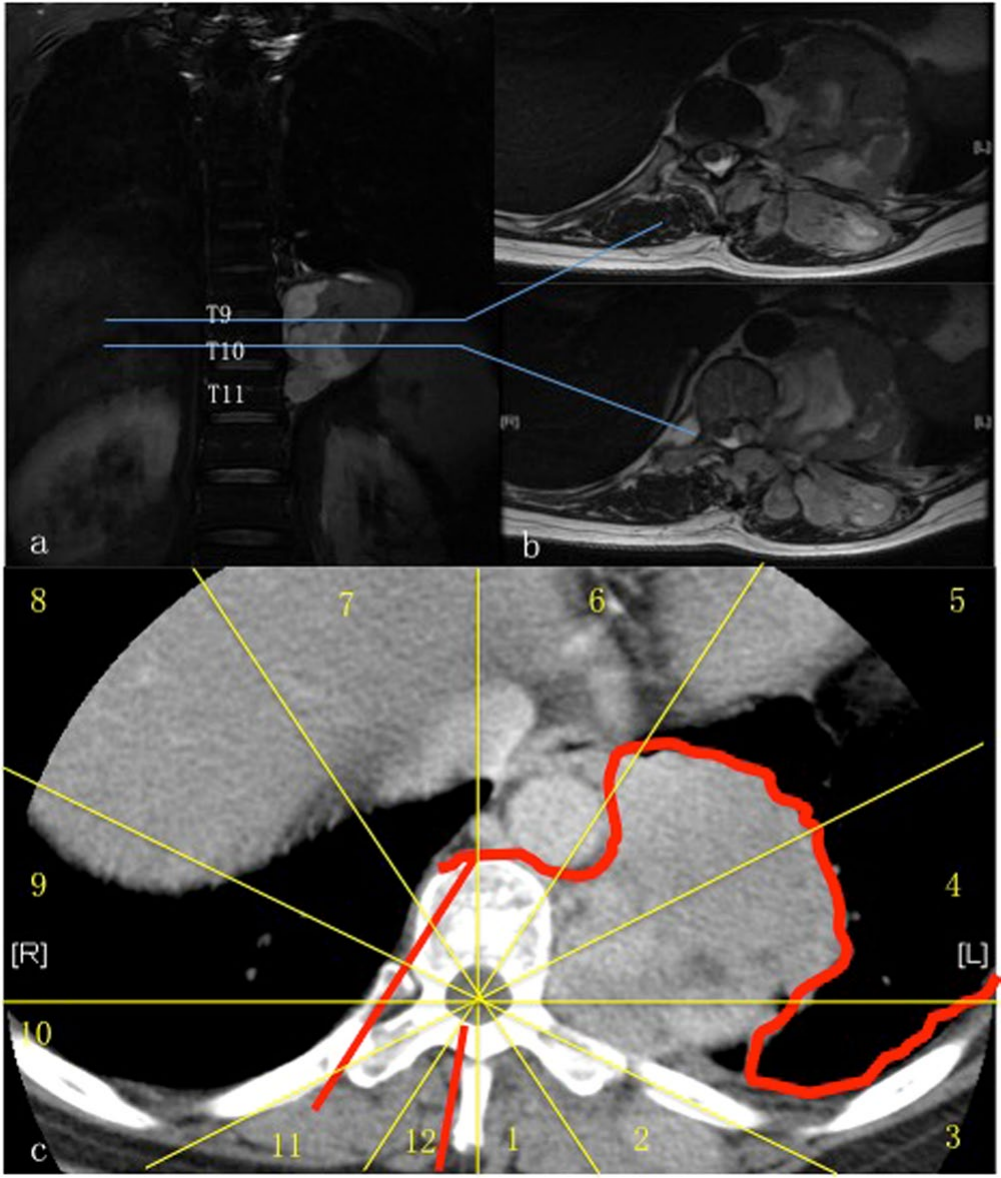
A case of a 63-year-old female with solitary fibroma at T9–11. The patient complained of numbness in the back and the abdomen 7 years after undergoing a piecemeal resection of a primary tumor in the paravertebral region of the lower thoracic spine in another hospital. Postoperative pathological diagnosis after initial surgery was solitary fibroma. a-c. MR images (a-b) and CT scans (c) show tumor recurrence along the left side of T9 to T11 vertebra (zone 1–5, A-D, WBB). Sagittal en bloc resection was planned based on WBB classification along the margin as highlighted in red.
